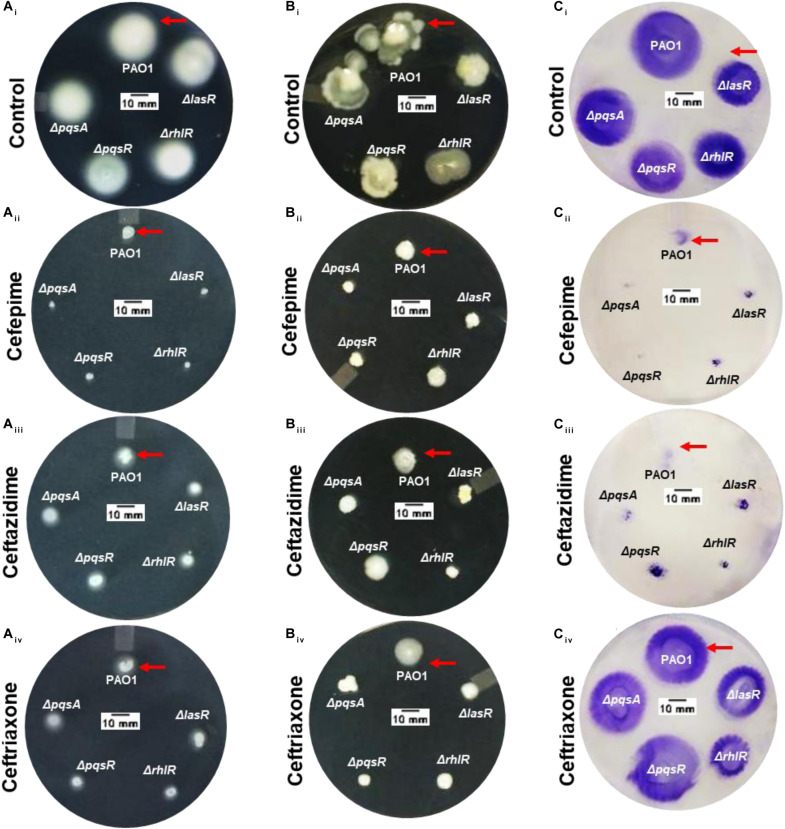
Effect of cephalosporins on motility phenotypes of *Pseudomonas aeruginosa* PAO1 and its isogenic QS mutant strains. Image showing the comparative zone of swimming (A_i_), swarming (B_i_), and twitching motility (C_i_) of PAO1 and its isogenic QS mutant strains (Δ*lasR*,Δ*rhlR*,Δ*pqsA*, and Δ*pqsR*). Image of the media plate showing the effect of CP (A_ii_,B_ii_,C_ii_), CF (A_iii_,B_iii_,C_iii_), and CT (A_iv_,B_iv_,C_iv_), on swimming, swarming, and twitching motility zones of *P. aeruginosa* PAO1 (Scale bar = 10 mm).
